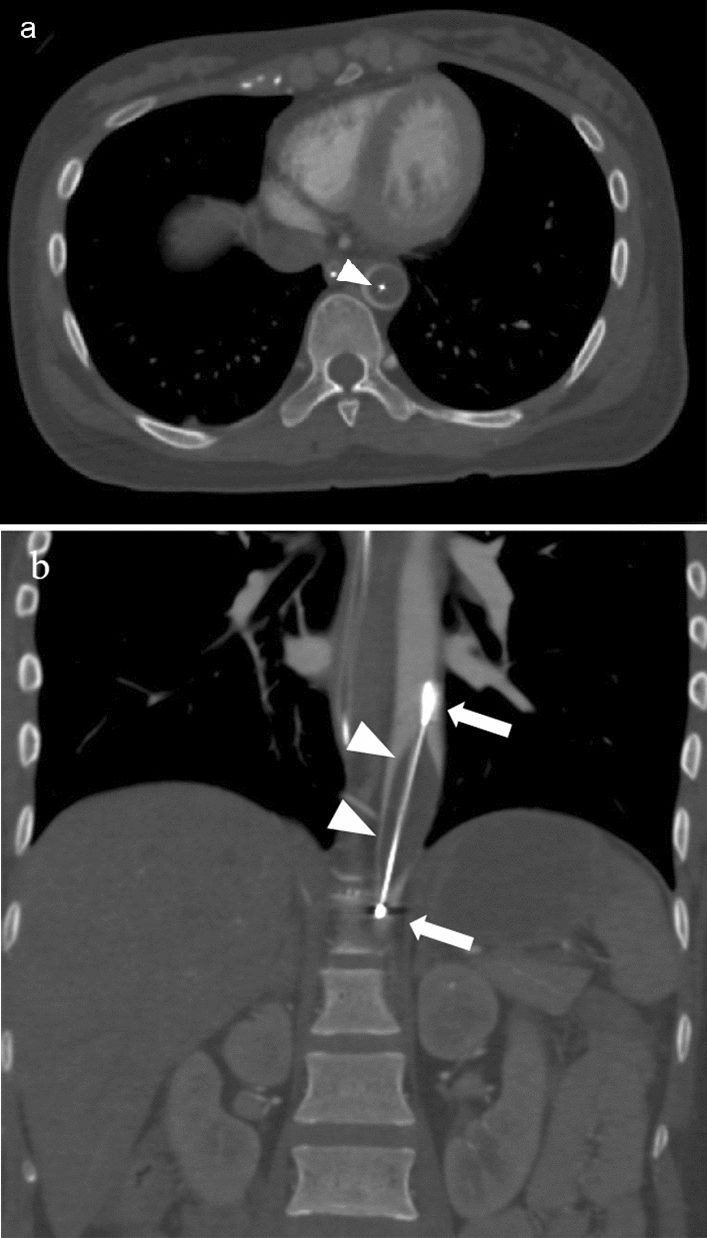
Contrast-enhancement CT (a: axial, b: coronal view) shows an appropriate partial resuscitative endovascular balloon occlusion of the aorta placed in Zone I. The white arrows represent radiopaque markers of the balloon catheter, and the white arrowheads represent the stylet in the balloon catheter
